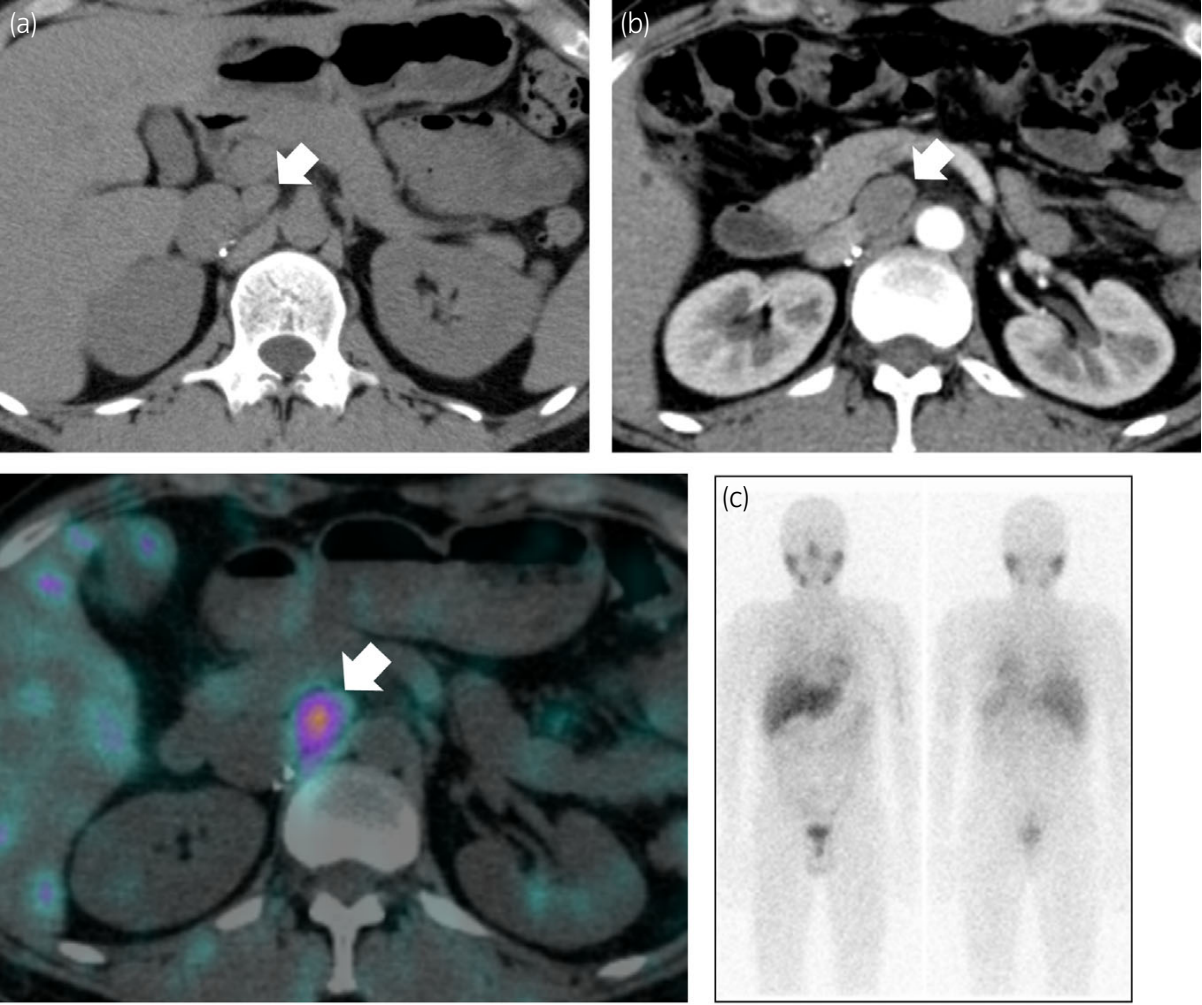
CT showing a tumor in the aortocaval area cranially to the renal vessels. The tumor size increased during follow‐up: (a) 14 × 9 mm in diameter in 2017 and (b) 25 × 18 mm in diameter in 2019. (c) ^123^I‐MIBG SPECT (left) and scintigraphy (right) in 2019. Arrows: tumor.
